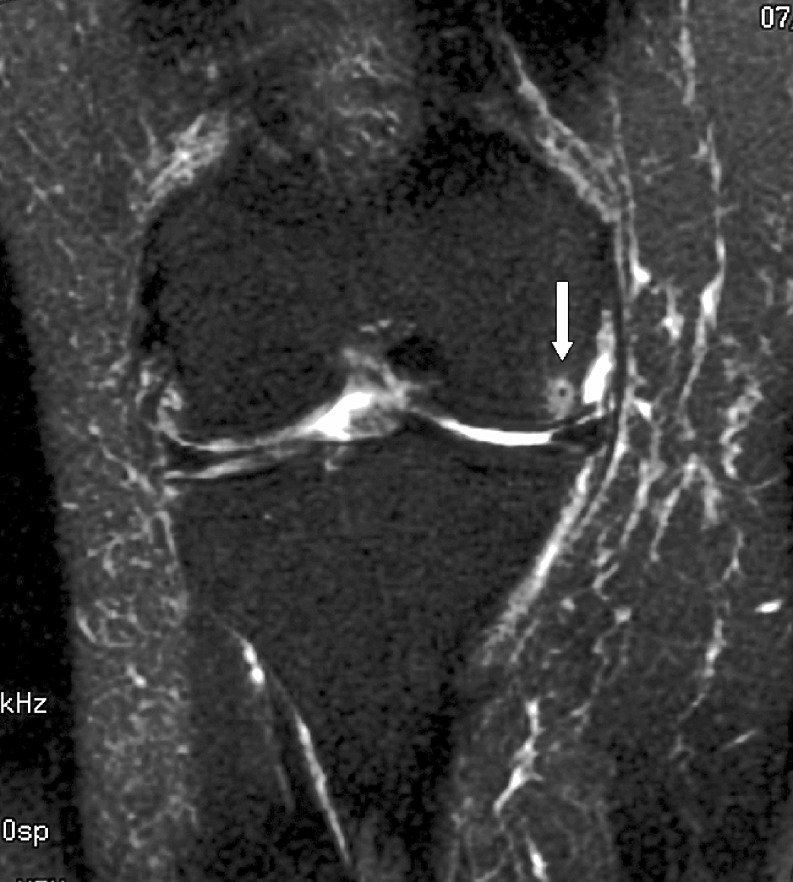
Focal edema with a total knee joint replacement in a 51-year-old female patient. The radiograph demonstrated moderate loss of cartilage joint space medially and spurring of the tibial spines. Coronal fast fat suppressed T2-weighted image (TR 3000/TE 76/256 × 192) shows the loss of hyaline cartilage and a small focal area of edema in the medial aspect of the medial femoral condyle (*arrow*)
